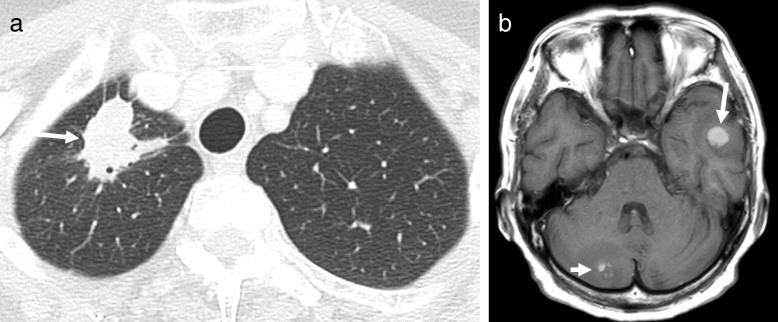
Radiological findings (July 2016). a Chest computed tomographic scan showing a tumor (3.1 cm) in the upper lobe of the right lung (*arrow*). b T1-weighted transverse magnetic resonance imaging of the brain showing a tumor in the left temporal lobe (*arrow*) and a tumor in the right cerebellar hemisphere (*short arrow*)
